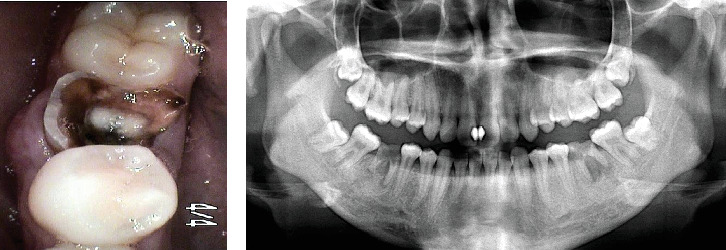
(a) A clinical view of the mandibular right first molar at the first examination. (b) A panoramic radiograph at the first examination.
